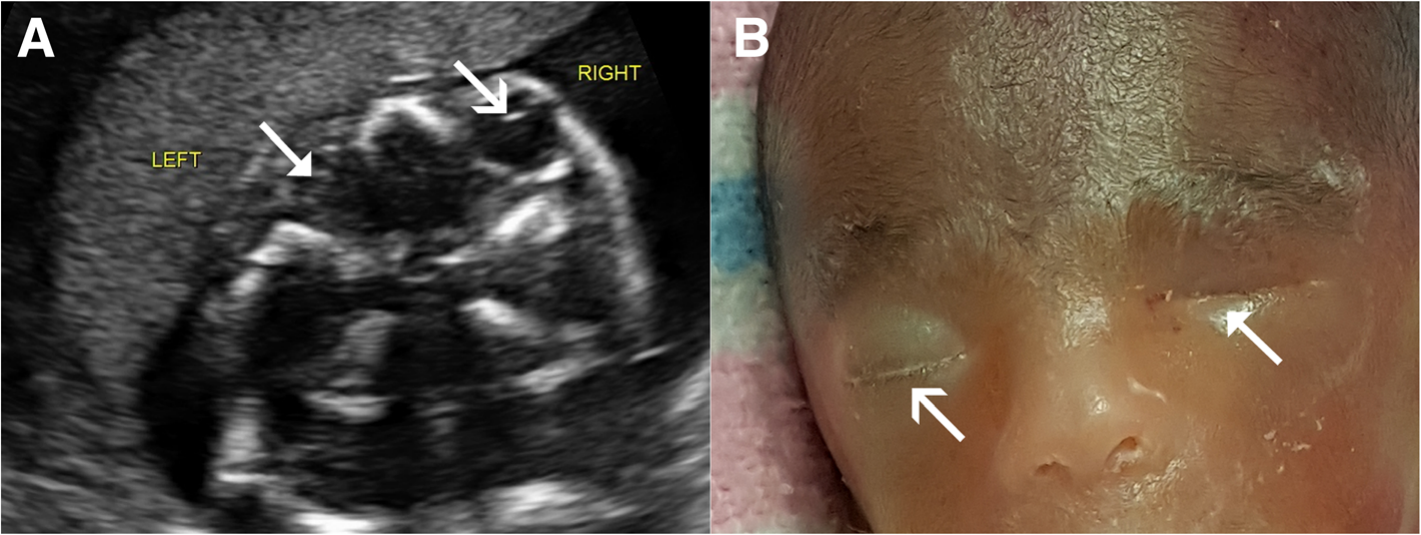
Case A. a Ultrasound findings of microphthalmia. b Postnatal view showing left-sided microphthalmia. *Triangle arrow-head* represents microphthalmia. The *simple wide arrow-head* represents the normally formed eye
